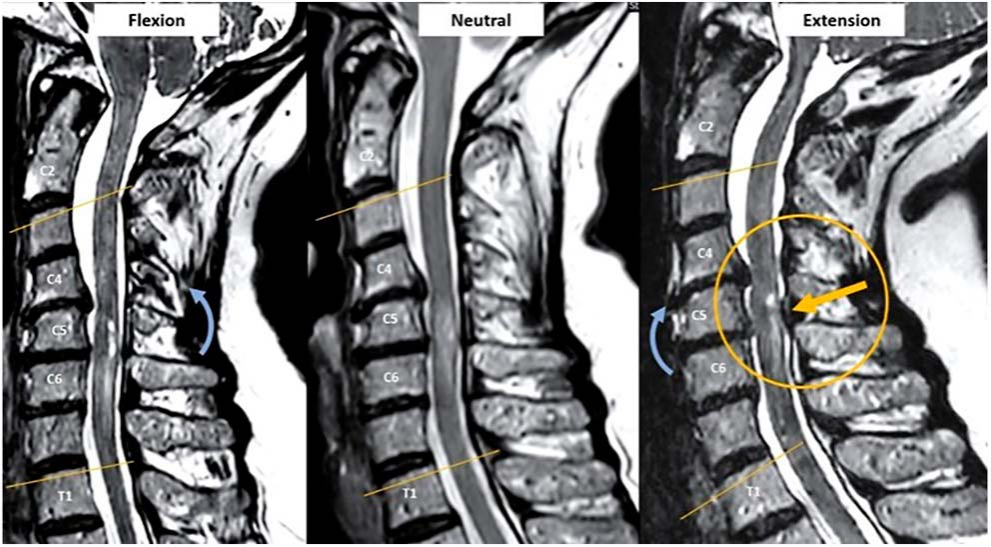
Dynamic MRI revealing severe spinal cord compression in extension at C5-C6, not clearly visible on neutral MRI.
